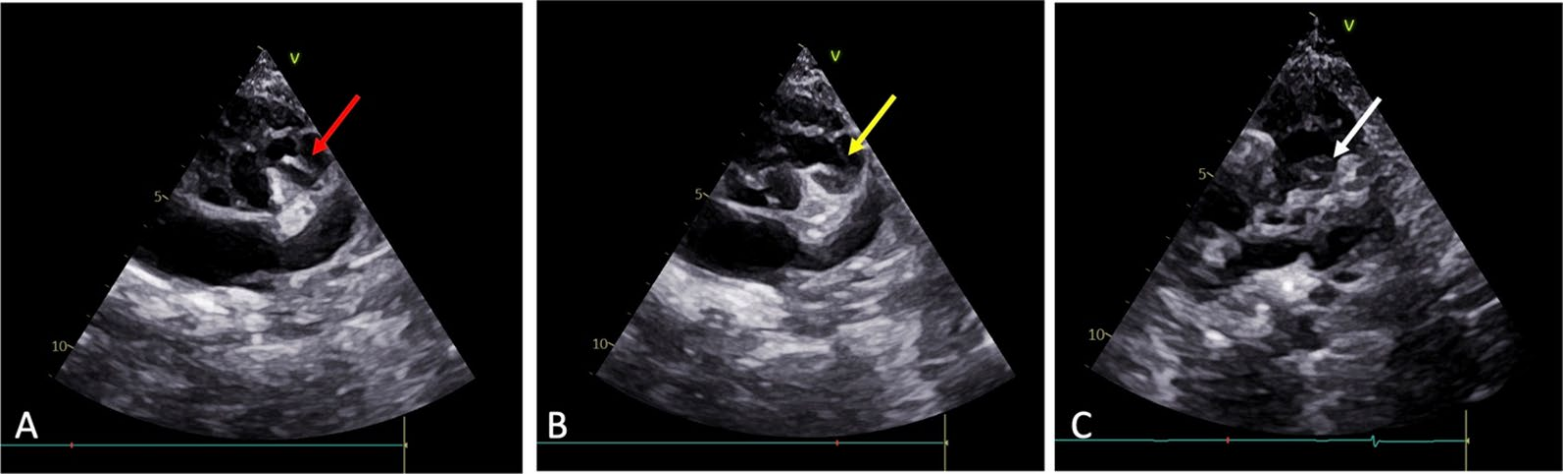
Modified Parasternal short axis view at level of aortic valve showing bicuspid pulmonary valve in Closed position (A, red arrow), doming valve in open position (B, yellow arrow) and horizontally oriented valve (C, white arrow)
